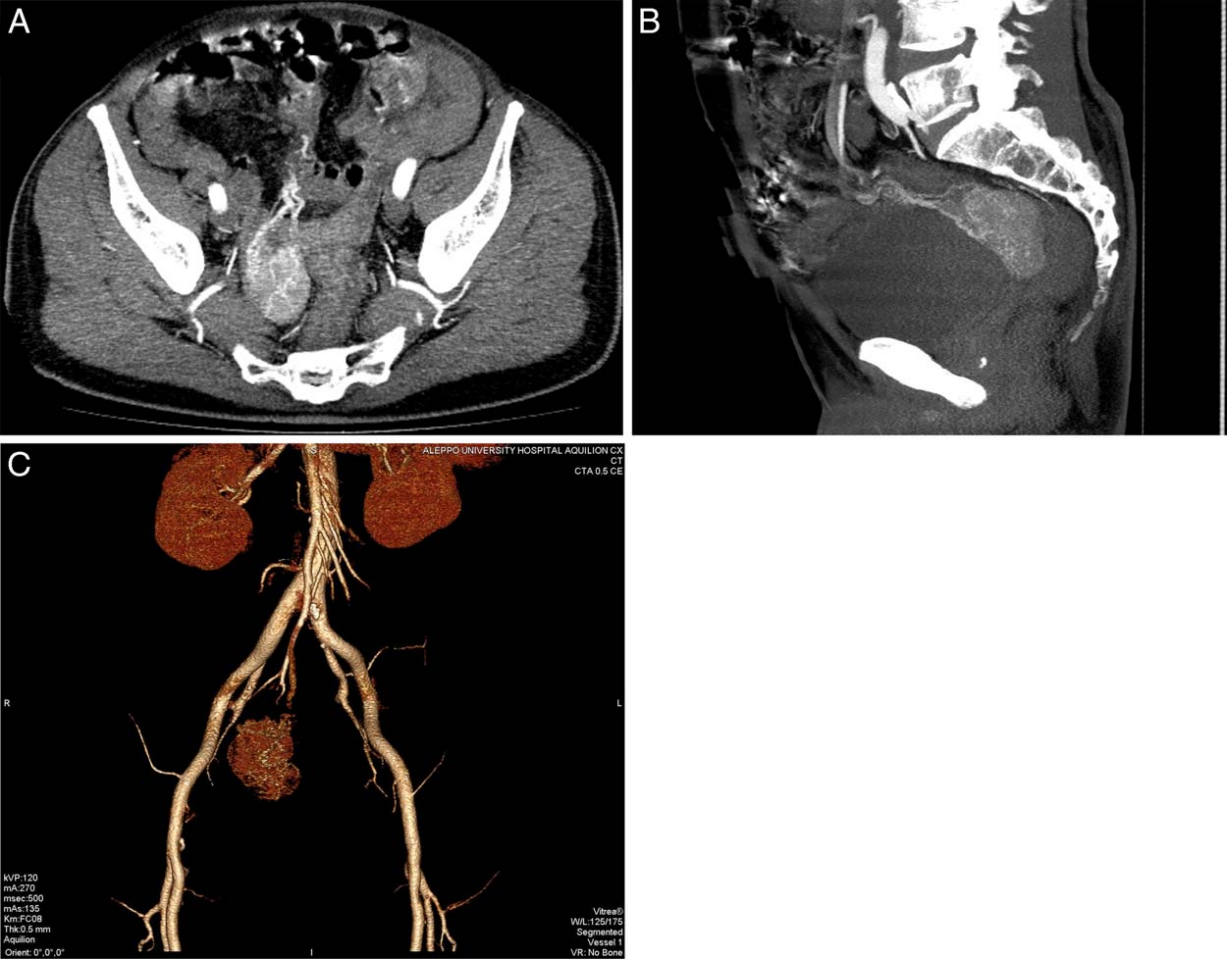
Contrast-enhanced computed tomography findings. (A, B) Axial and sagittal views in the arterial phase showcasing a well-defined hyperdense extraluminal mass in the terminal ileum situated in the retrovesical pouch. (C) Three-dimensional reconstruction of computed tomography angiography in the arterial phase showing abnormal arterial neovascularization from the superior mesenteric artery to the ileal mass.
